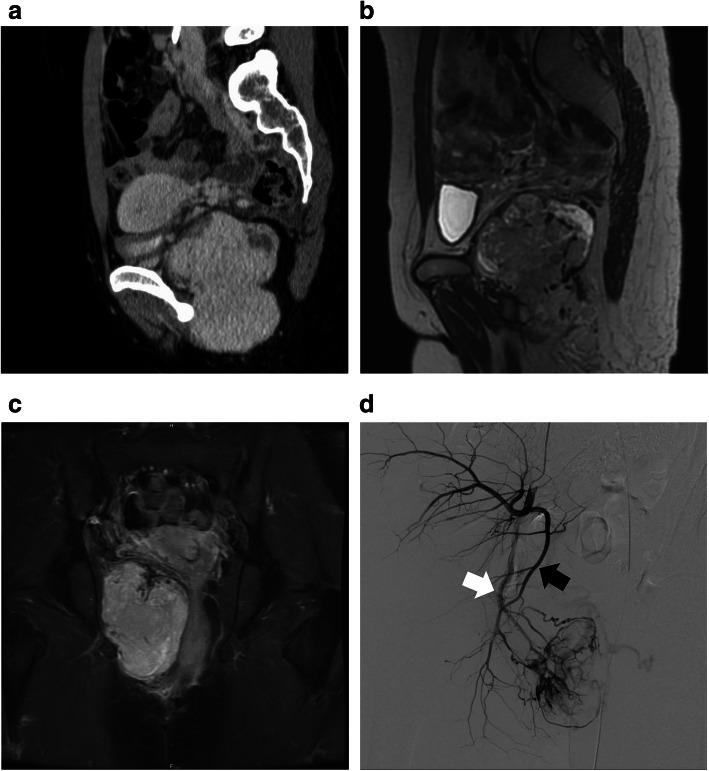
Preoperative imaging findings. a Preoperative contrast medium–enhanced sagittal computed tomography showed a pelvic mass lesion, measuring 112 × 62 × 58 mm. b T2-weighted sagittal magnetic resonance imaging (MRI) showed a mass with heterogeneous intensity in the pelvic cavity. c
